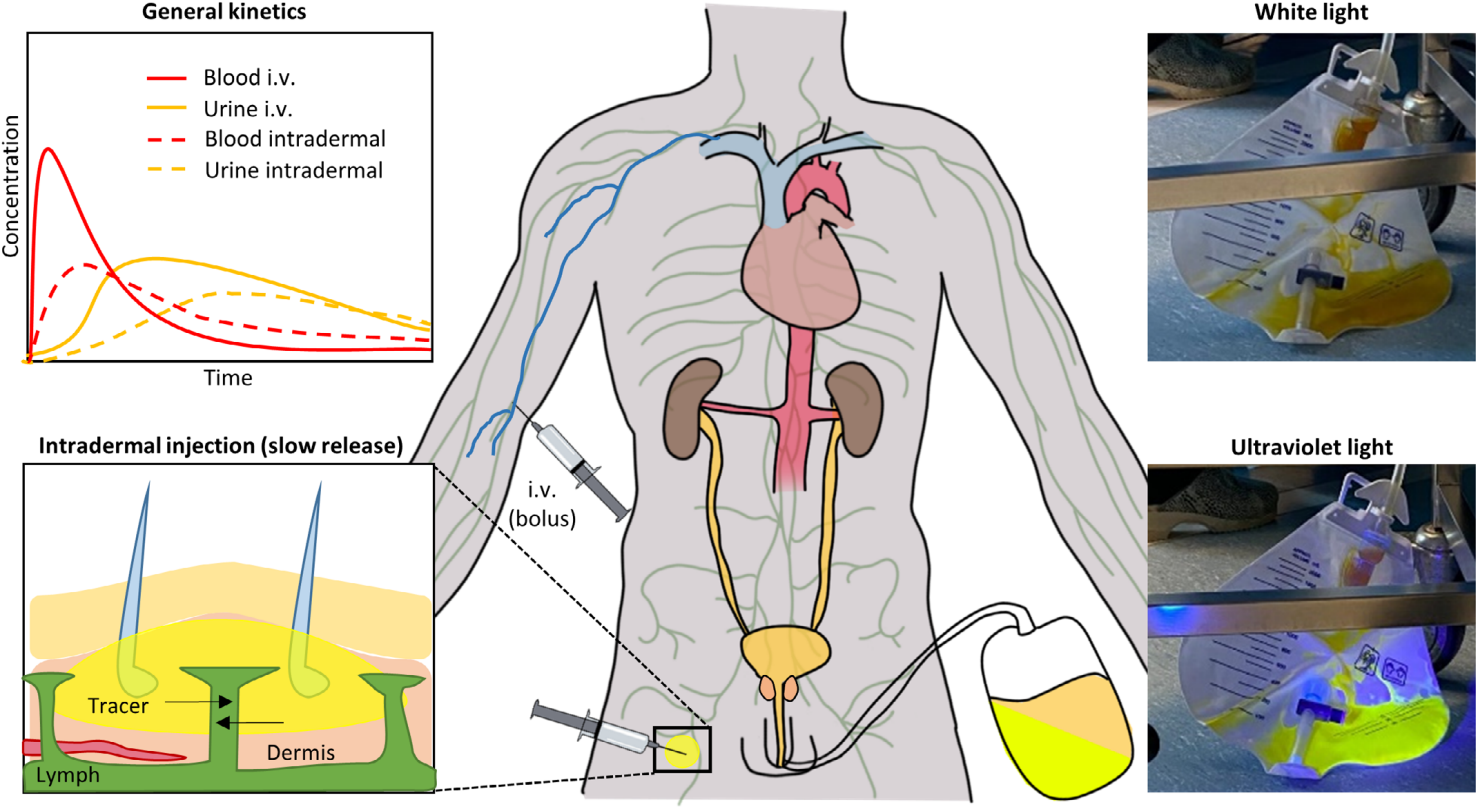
General pharmacokinetics based on ADME Encyclopedia and Buckle et al. [10, 33]. Intravenous injection facilitates immediate presence of agents in the (early peak). Intradermal injection creates a more stationary agent deposition at the site of injection, followed by slow release from the tissue. As a result, the latter can be used to mimic receptor‐targeted agents, such as PSMA‐targeted agents, that remain available over a prolonged period of time. As fluorescein is renally cleared, it was visible in the urine in the bedside catheter bag using ultraviolet flashlight.
